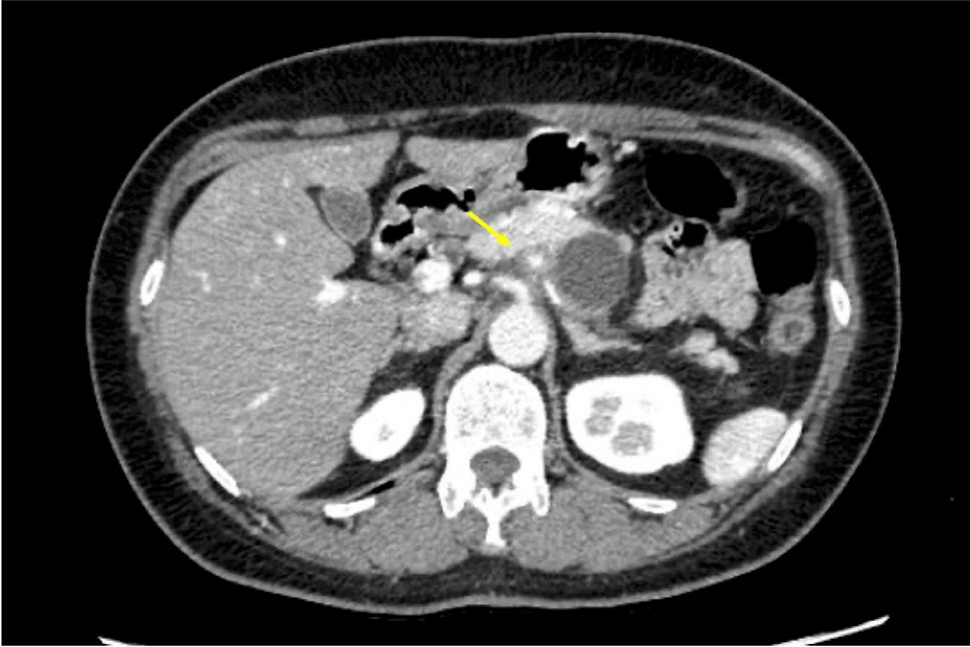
Enhanced computed tomography revealed a large 3-cm unilocular cystic lesion in the pancreatic body and a soft tissue lesion around the splenic artery on the papillary side (yellow arrow)
